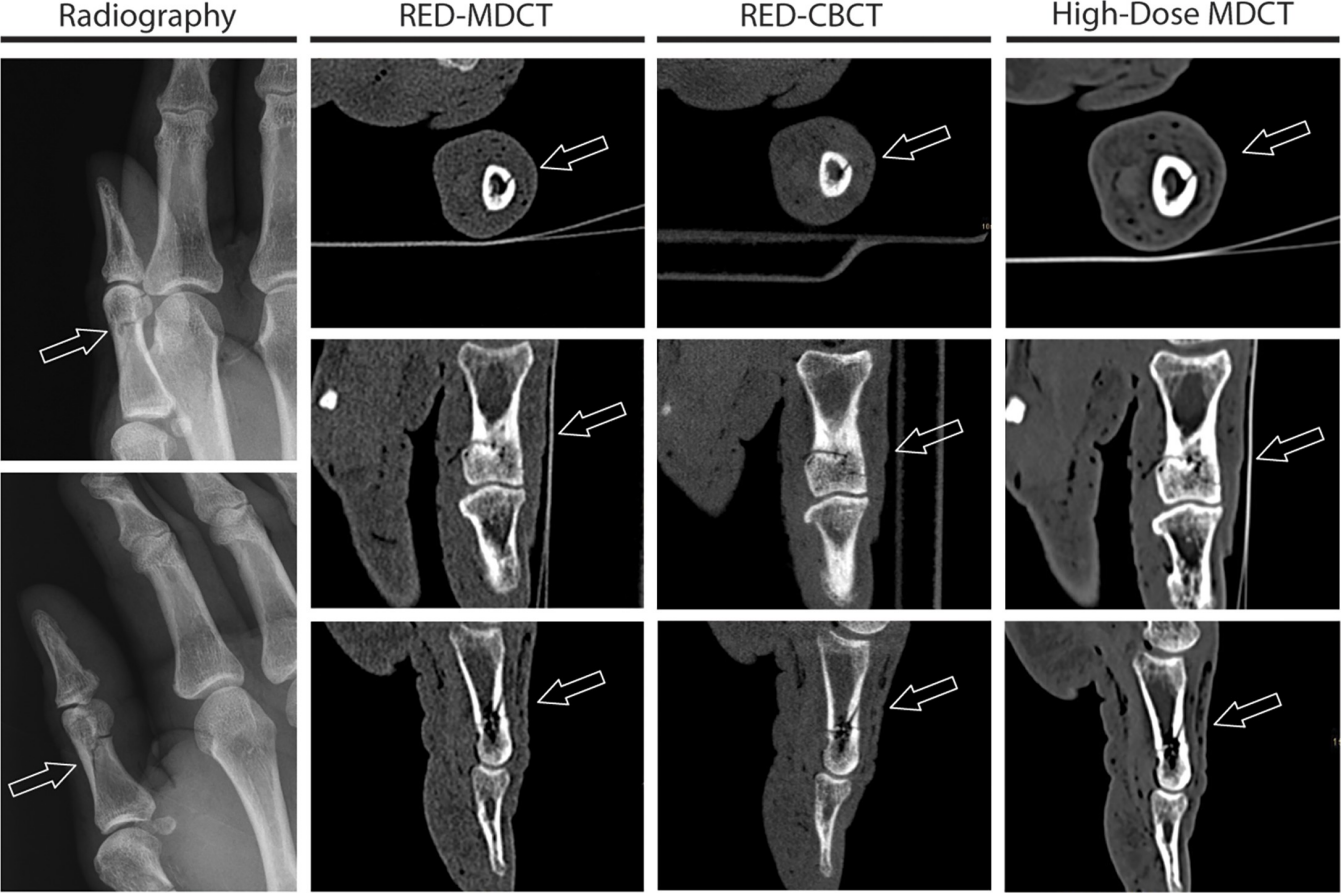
Fracture of Digitus I, Phalanx proximalis (see arrow pointing towards fracture)—Imaging examples with axial, coronal and sagittal reconstructions (top down) for RED-MDCT (second column), RED-CBCT (third column), high-dose MDCT (fourth column) and radiography (first column—p.a., top; and oblique, bottom).
